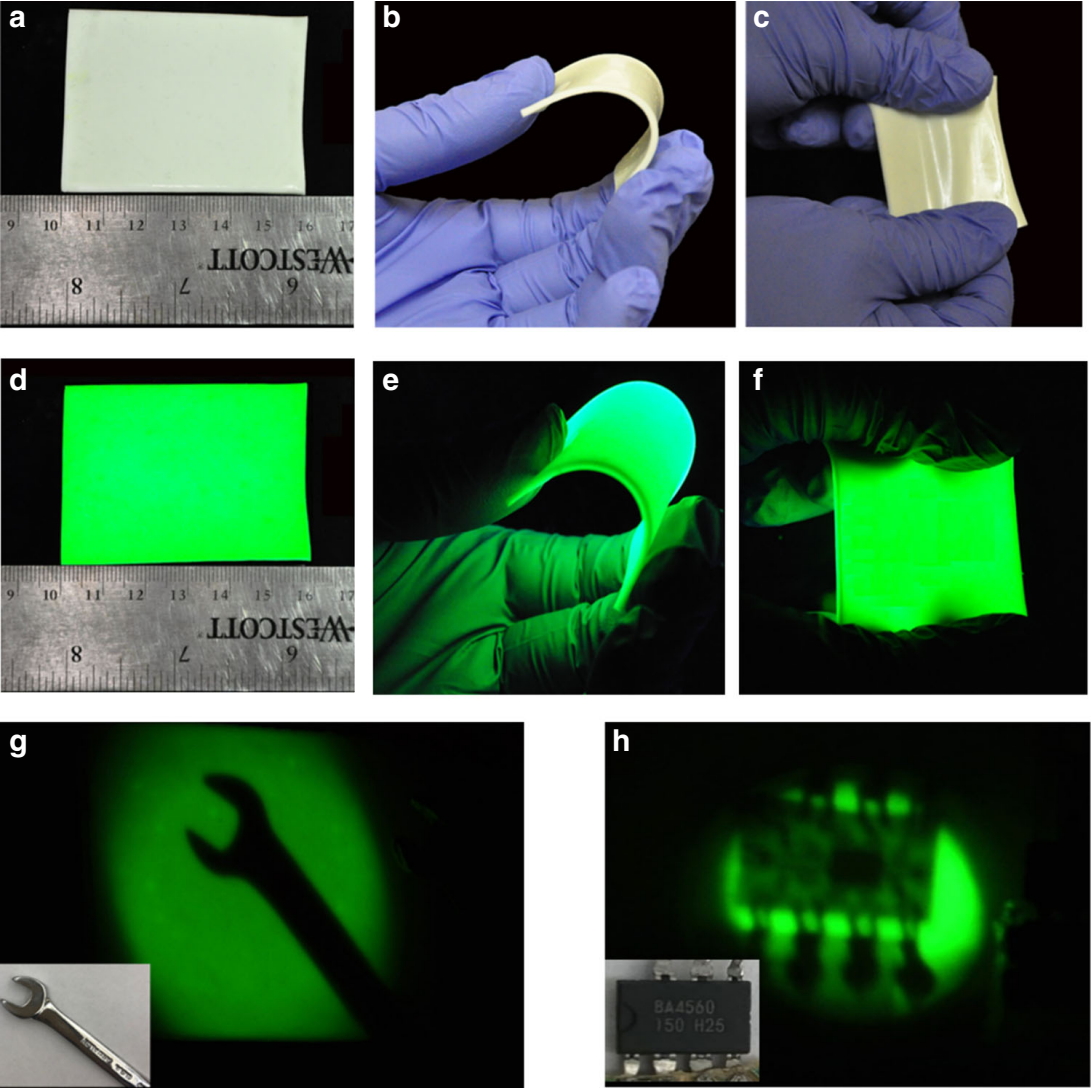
Flexible X-ray scintillator screens. The photographs of a flexible scintillator screen based on (C_38_H_34_P_2_)MnBr_4_
